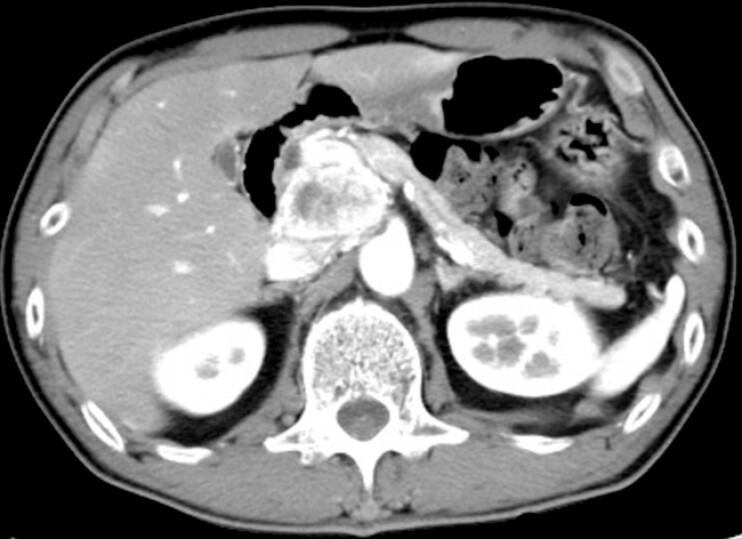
Computed tomography image of a retroperitoneal paraganglioma. The tumor was located adjacent to the head of the pancreas.
